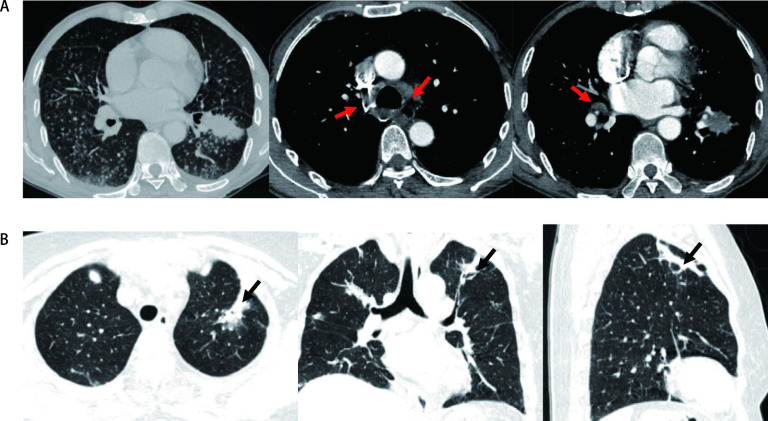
患者胸部CT检查。A：肺部不规则高密度影，伴有纵隔淋巴结肿大；B：于左肺上叶高密度不规则肿物影处行CT引导下经皮肺穿刺活检。红色箭头：肿大的纵隔淋巴结；黑色箭头：经皮肺穿刺活检的穿刺点。 CT scans of the chest. A: The chest CT indicating the irregular high-density shadows of the lung. There were amount of irregular infiltration shadow volume around and mediastinal lymph node enlargement; B: An image-guided percutaneous transthoracic needle biopsy (PTNB) is performed on the mass in the upper lobe of the left lung. Red arrow: Mediastinal lymph node enlargement; black arrow: The point for percutaneous lung biopsy. CT: computed tomography.
